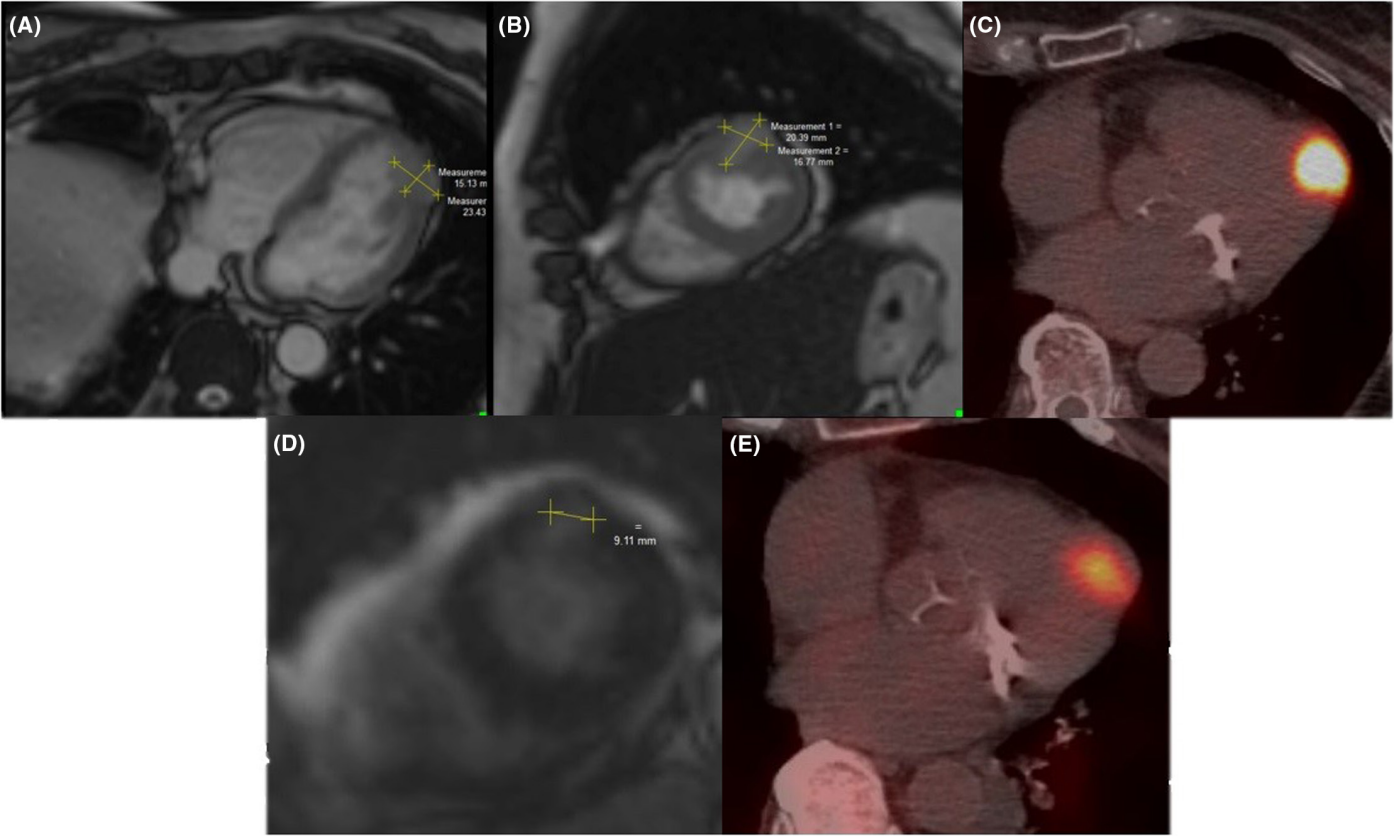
Imaging of a patient with metastatic pNET to the heart, treated with [177Lu] Lu‐DOTA‐TATE and lanreotide. (A, B) Cardiac magnetic resonance imaging (cMRI) with gadolinium (Gd) contrast showing our patient's cardiac metastases prior to treatment. The mass measured 15 mm x 23 mm and did not impact cardiac function (ejection fraction was estimated as 75%). Short axis (A) and axial (B) orientations are shown. (C) Positron emission tomography (PET) imaging with gallium (Ga)‐68 contrast taken prior to patient treatment showing hyperintensity at the same location as the mass seen in Figure 1A, B; intensity was measured at 27.4 standardized uptake units (SUV). (D) cMRI showing decreased lesion size compared to Figure 1A, B (now about 9 mm in diameter at widest point). (E) PET imaging after treatment with [177Lu] Lu‐DOTA‐TATE showing decreased uptake (now 24.8 SUV) compared to Figure 1C.
